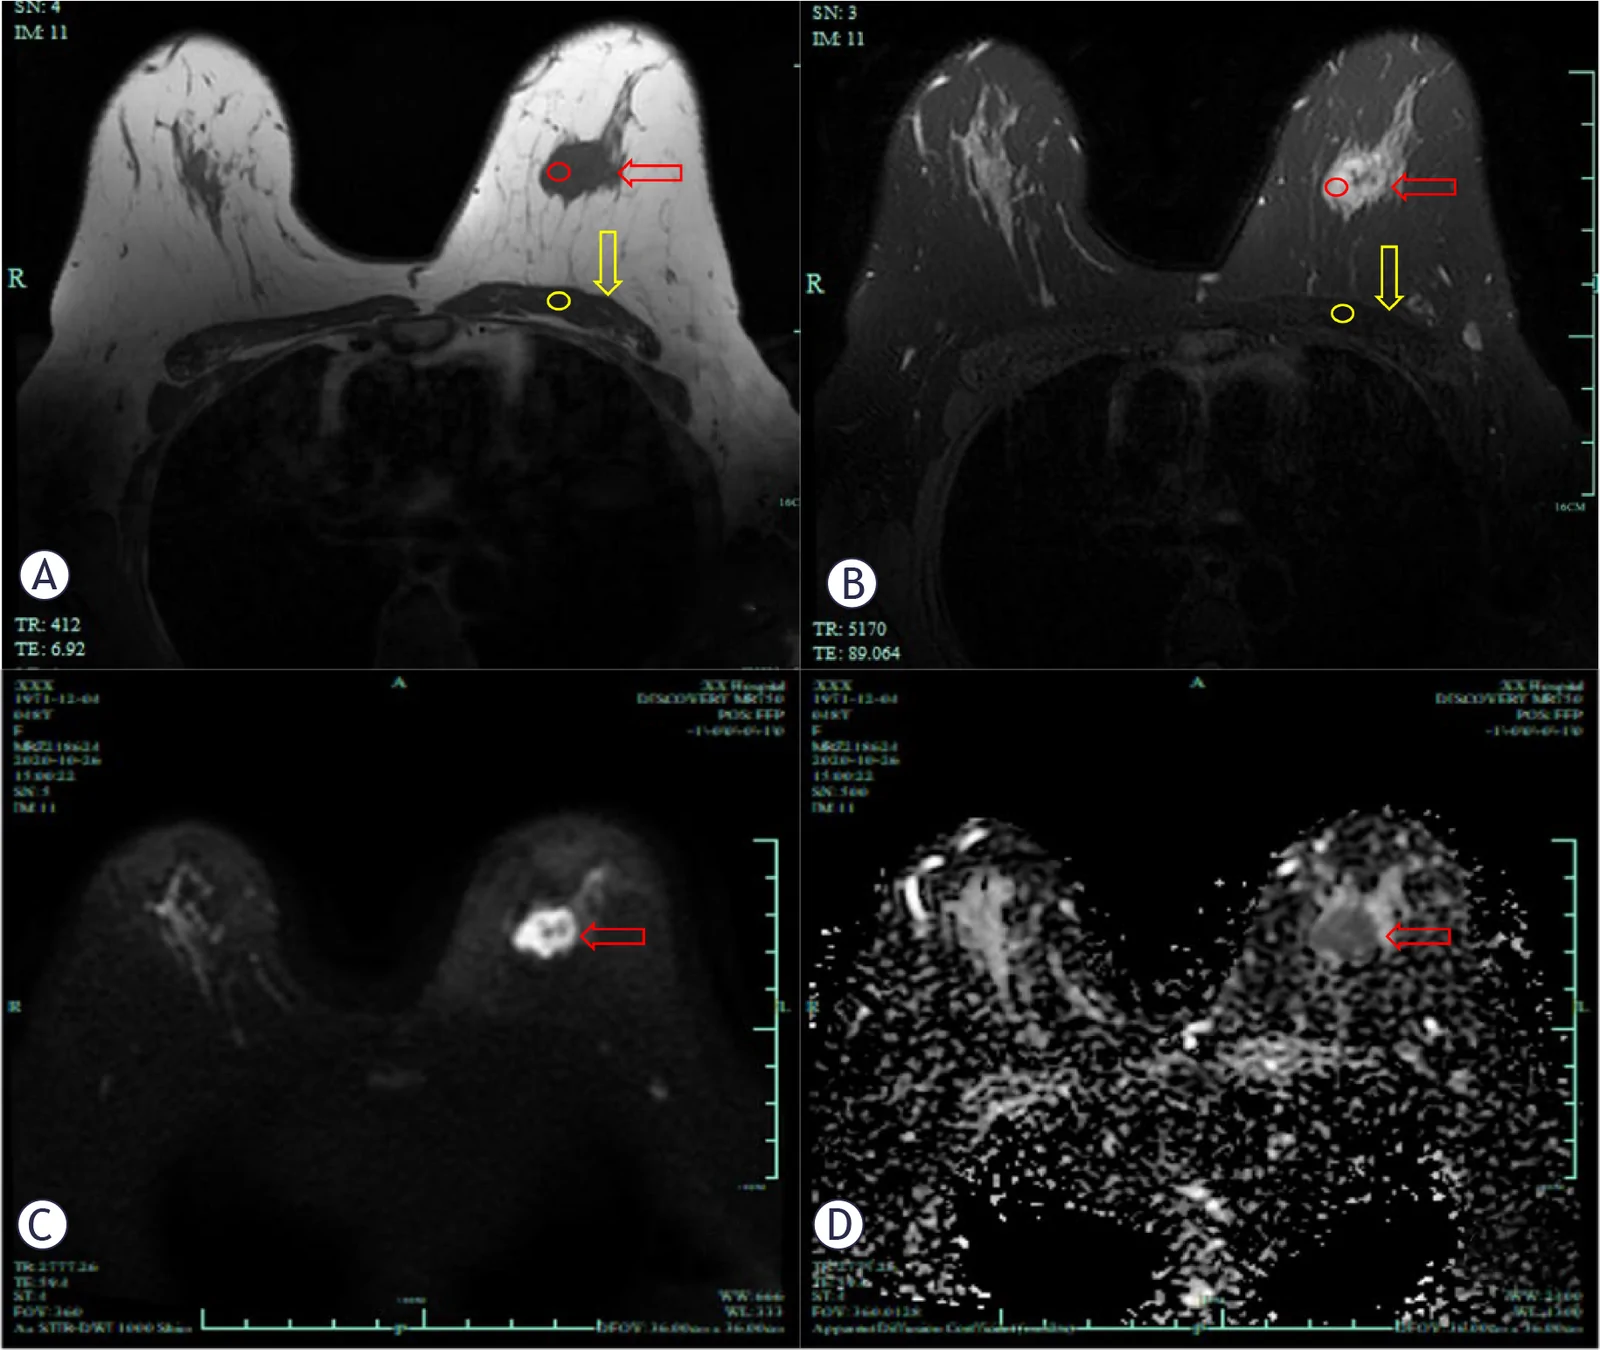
MR images of a 48 years-old patient diagnosed with luminal B subtype breast cancer. T1 weight image (A), T2 fatsuppression sequence image (B), DWI image (C) and ADC map (D) was shown. T1 and T2 signal intensity ratio (A and B) were calculated by proportioning the average signal intensity of the lesion (red circle) to the average signal intensity of the pectoral muscle (yellow circle). Red arrows show the lesions and yellow arrows show the pectoral muscles. ADC = apparent diffusion coefficient; DWI = diffusion-weighted imaging
